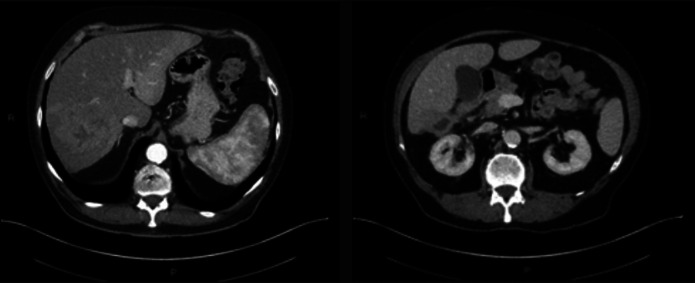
Arterial phase computed tomography demonstrated a large heterogenous mass in the right lobe of the liver measuring up to 11.2 cm (predominantly in segment 7 or 8). Areas of solid enhancement as well as a central necrosis are present. IgG4-related pseudotumors usually present with variable enhancement patterns, most commonly exhibiting homogenous delayed enhancement.
